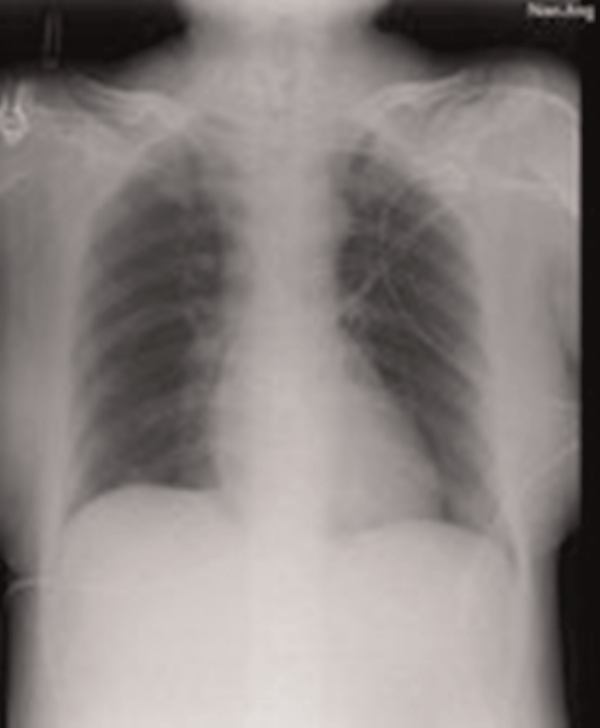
Chest X-ray film (25 days after on admission of ICU). Pneumonia was cured.
